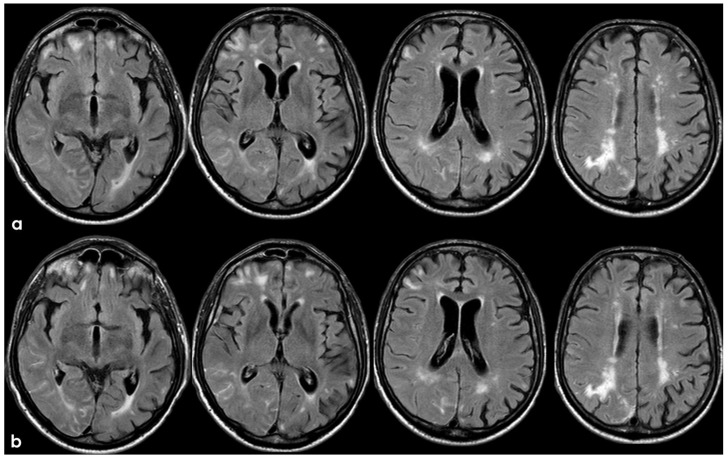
Brain MRI with axial FLAIR before (panel (a)) and after (panel (b)) contrast administration. In panel (a), a diffuse cortical swelling in the right temporal-occipital, frontal, and parietal lobes was evident, associated with subarachnoid hyperintensity in almost all examined sulci, and parenchymal white matter hyperintensity was evident in the right cingulus and in the right anterior frontal lobe (this was more extended than in previous MRIs). All hyperintense sulci in panel (a) were contrast-enhanced in panel (b).
